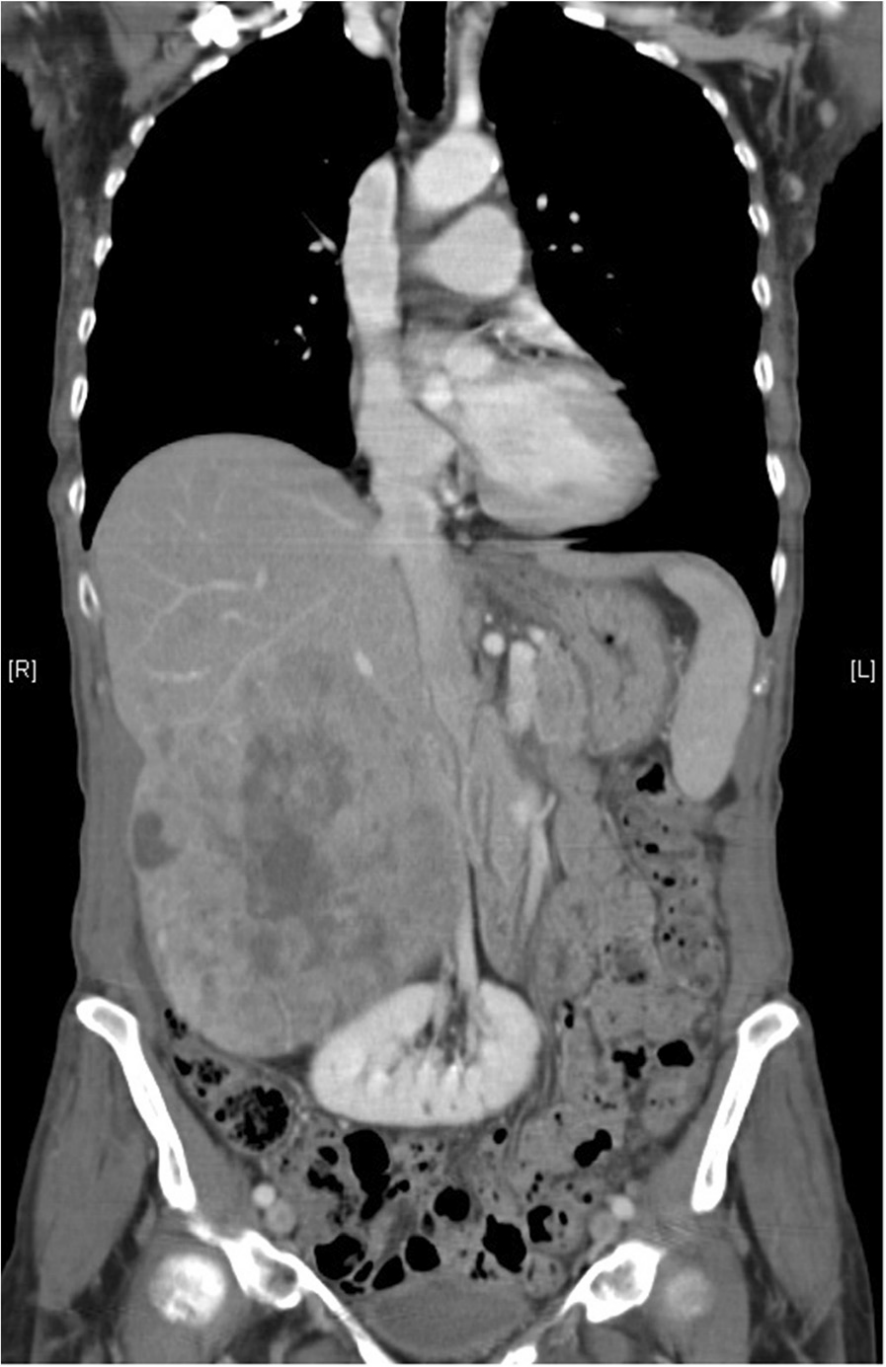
Computed tomography at initiation of pembrolizumab. Computed tomography scan of the liver before treatment with pembrolizumab was initiated after progression with the treatment of ipilimumab.
